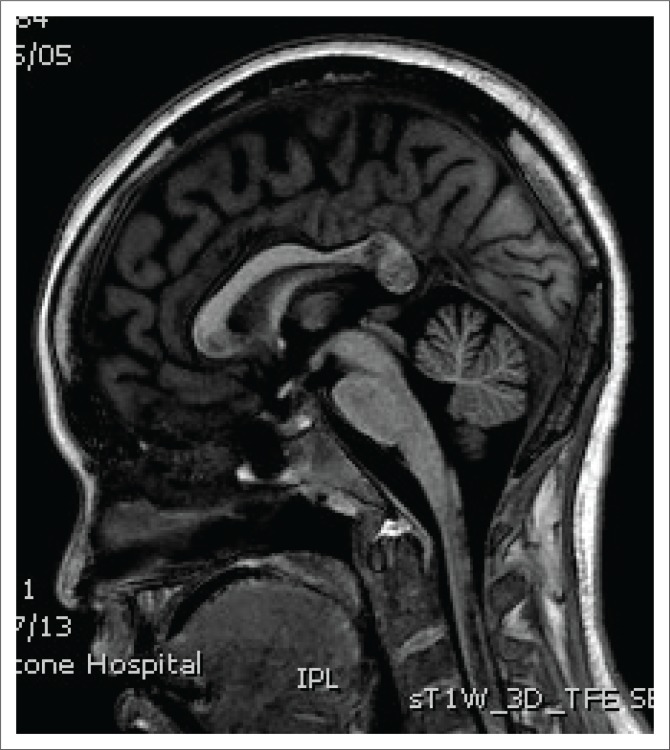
Sagittal T1-weighted image depicting hypointensity in the splenium and cervical spinal cord.
